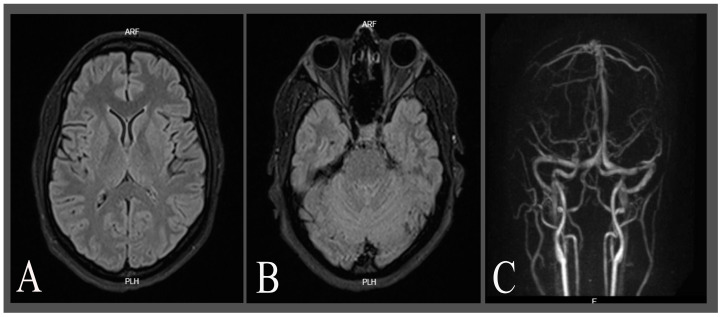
Non-enhanced brain MRI and time-of-flight magnetic resonance angiography (TOF-MRA). (A,B) show fluid attenuating recovery time (FLAIR) sequences showing intact grey and white matter structures with no signs of intracranial hypertension, evidence of lesions, haemorrhage, or other pathological abnormalities, and diffusion sequences. (C) shows a 3D reconstruction of a normal TOF-MRA.
